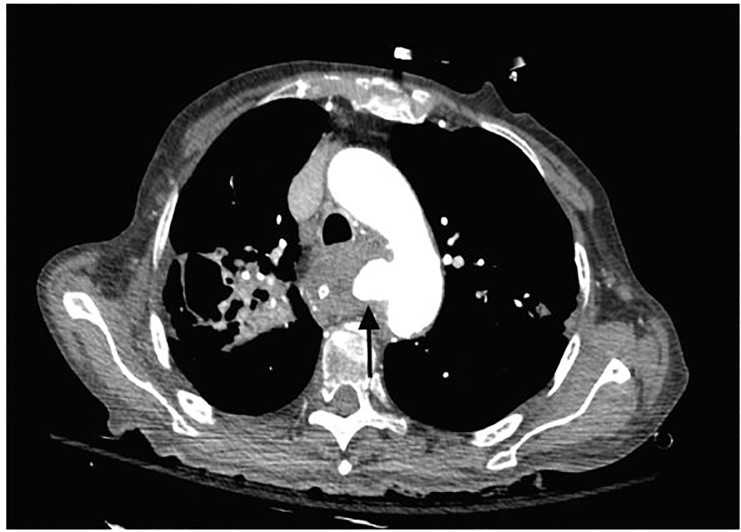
Computed tomography of the chest with contrast-filled aortoesophageal fistula (arrow).
